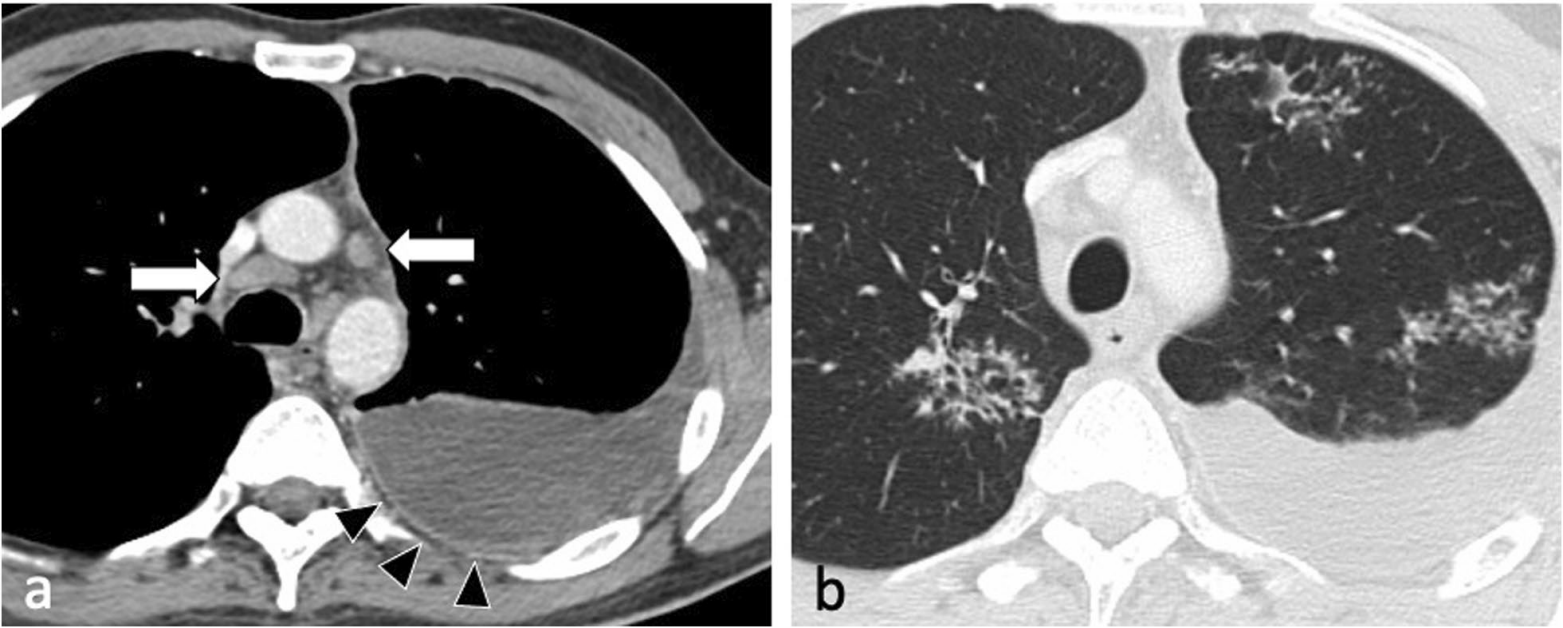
A 37-year-old male presented with intermittent pyrexia, sweats, rigors, reduced appetite and weight loss. Post-contrast CT shows a moderate volume left pleural effusion with pleural thickening and enhancement (mediastinal window, axial plane—a, arrowheads). There are also multifocal areas of perilymphatic nodularity (lung window, axial plane—b) and bilateral paratracheal and mediastinal lymphadenopathy (arrows, a). Pleural biopsy confirmed the presence of acid-fast bacilli
